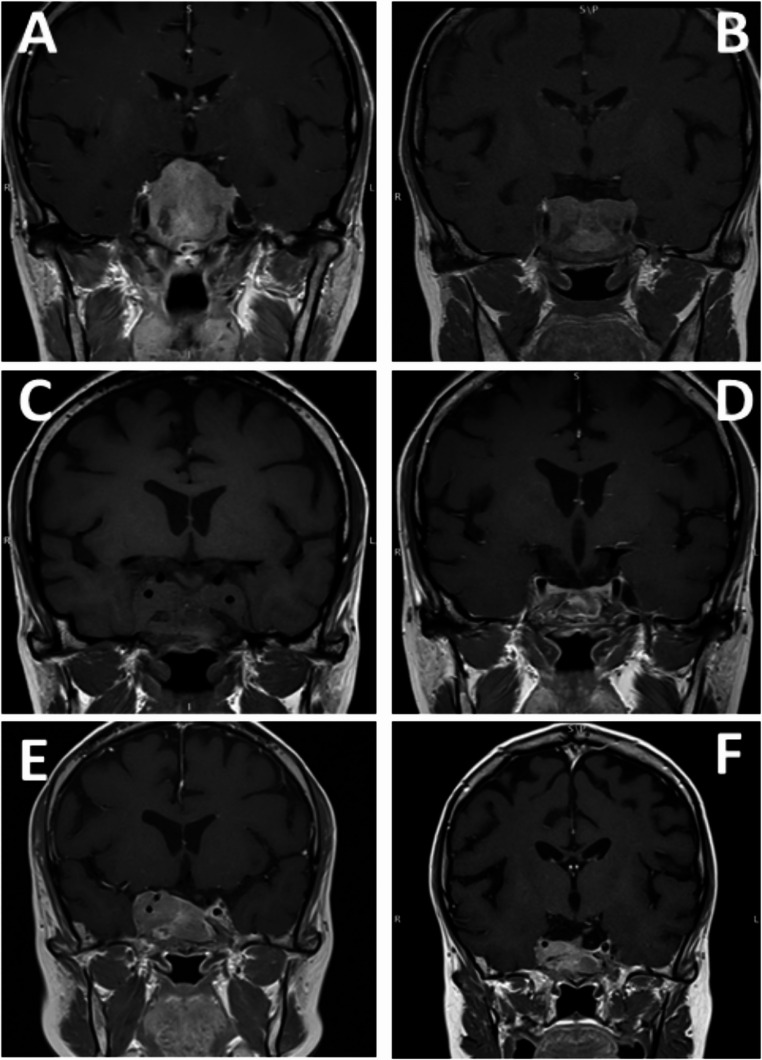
Magnetic resonance images of patient n. 5 Magnetic resonance imaging (MRI) in T1 weighted sequences of patient n. 5 showing tumor lesion after BADX prior to TSS (Panel A); tumor debulking after TSS (Panel B); tumor re-growth 12 months post-TSS and prior to medical treatment (Panel C). Tumor shrinkage following treatment with TMZ plus pasireotide (Panel D). MRI 12 months after discontinuation of TMZ, showing tumor progression with invasion of the clivus and right cavernous sinus, along with initial narrowing of the carotid siphon (Panel E). Partial tumor regression after a second course of TMZ combined with SRS (Panel F)
